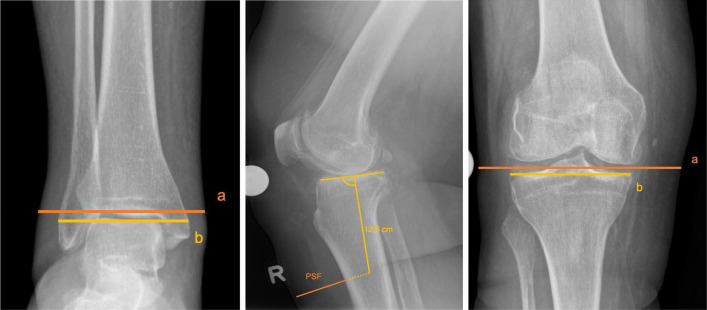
Measurement of the Local Soft Tissue Thickness (LSTT). Representative images demonstrating the measurement of LSTT parameters on preoperative radiographs of a right knee are presented from left to right. The ankle-adipose-index (AAI) was defined as the ratio of the total thickness of the ankle at the level of the malleoli (a) to the bony width of the ankle (b) (left image). The pretibial subcutaneous fat (PSF) is measured as the distance from the anterior cortex of the tibia to the overlying skin, using a perpendicular line drawn 12.5 cm distal to the tibial plateau (central image). The knee-adipose-Index (KAI) was calculated as the ratio of the total soft tissue thickness (a) to the width of the tibial plateau (b) (right image)
